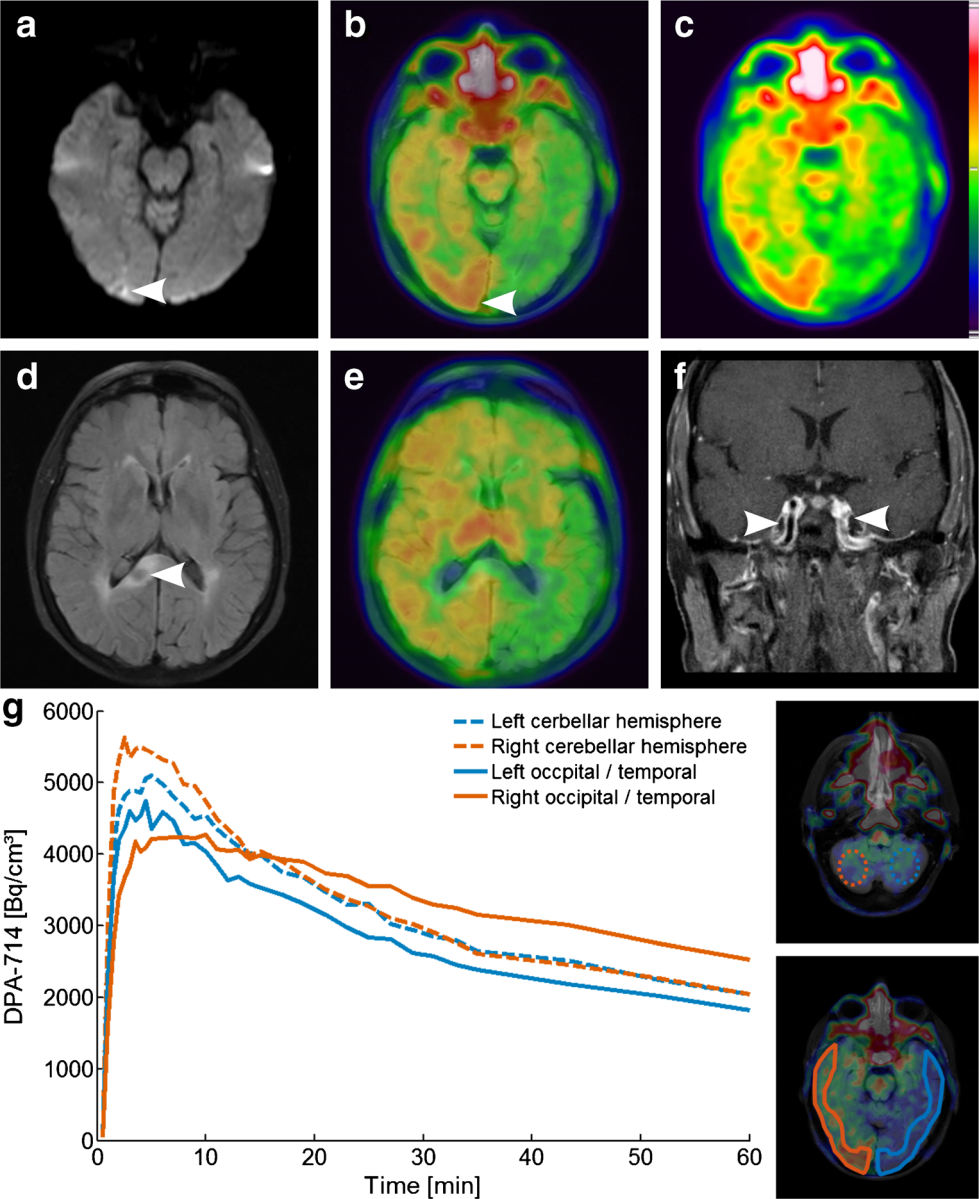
Imaging findings of patient #2 diagnosed with PACNS. [^18^F]DPA-714-PET images fused to FLAIR demonstrate elevated tracer uptake of the whole cerebral hemisphere. Right-sided occipital pole hotspot (b, arrow FLAIR/PET overlay, c, PET) corresponds to diffusion restriction as indicated by DTI (a, white arrow, MR scan 5 days before PET/MRI). FLAIR image of subacute ischemia in the right splenium (d, white arrow) corresponding to fusion image (e). Coronal T_1_wi after contrast agent application demonstrates vessel wall uptake (f, white arrows, contrast-enhanced MR scan 5 days before PET/MRI). (g) Time activity curves of symmetric ROIs on the occipital / temporal cortices and symmetrical spherical VOIs on the cerebellar hemispheres as indicated in the inset images
